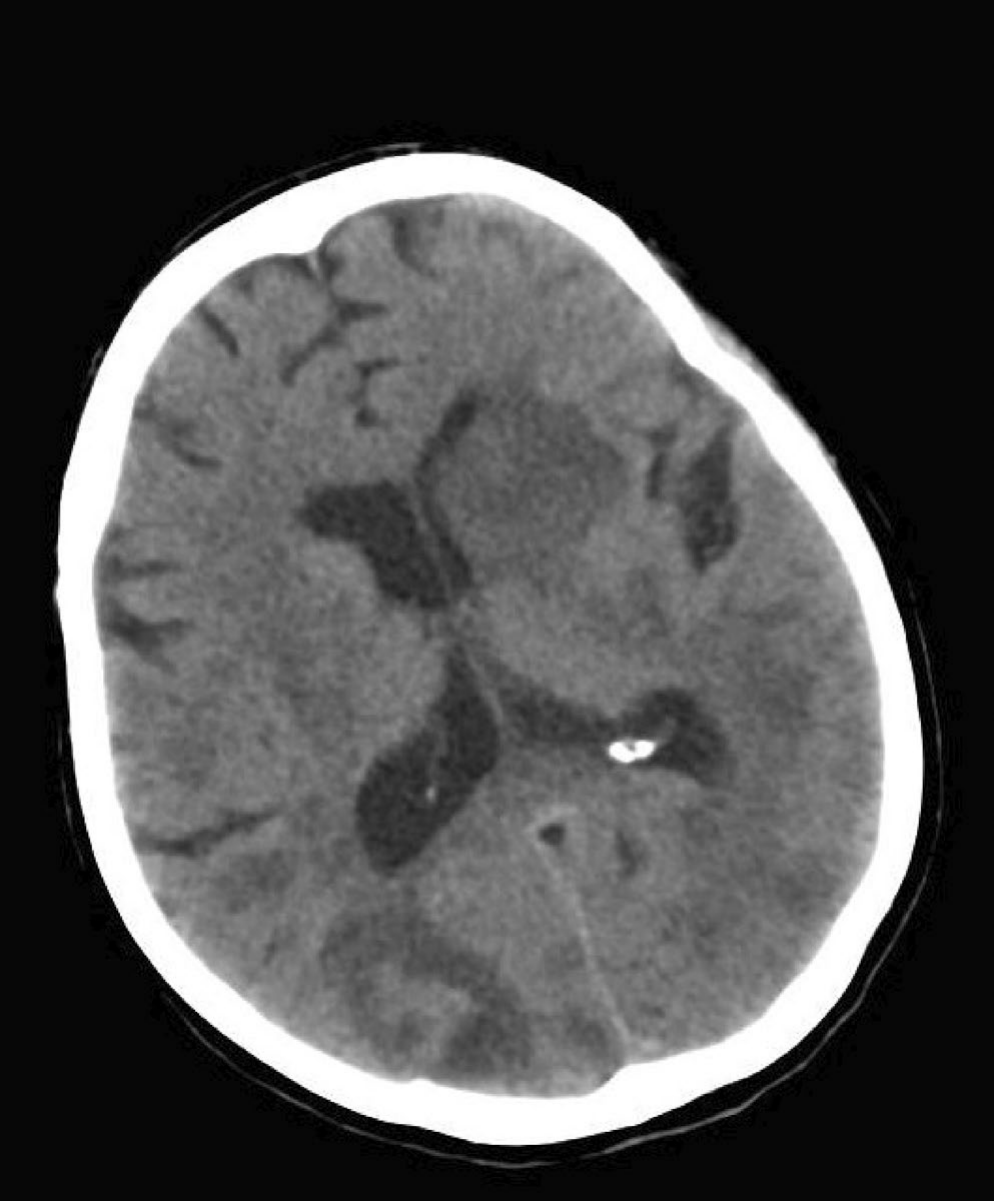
FIGURE 1 CASE 1 Noncontrast enhanced computed tomography demonstrating multiple hypodense lesions, most prominent in the left basal ganglia and right parietal lobe.
